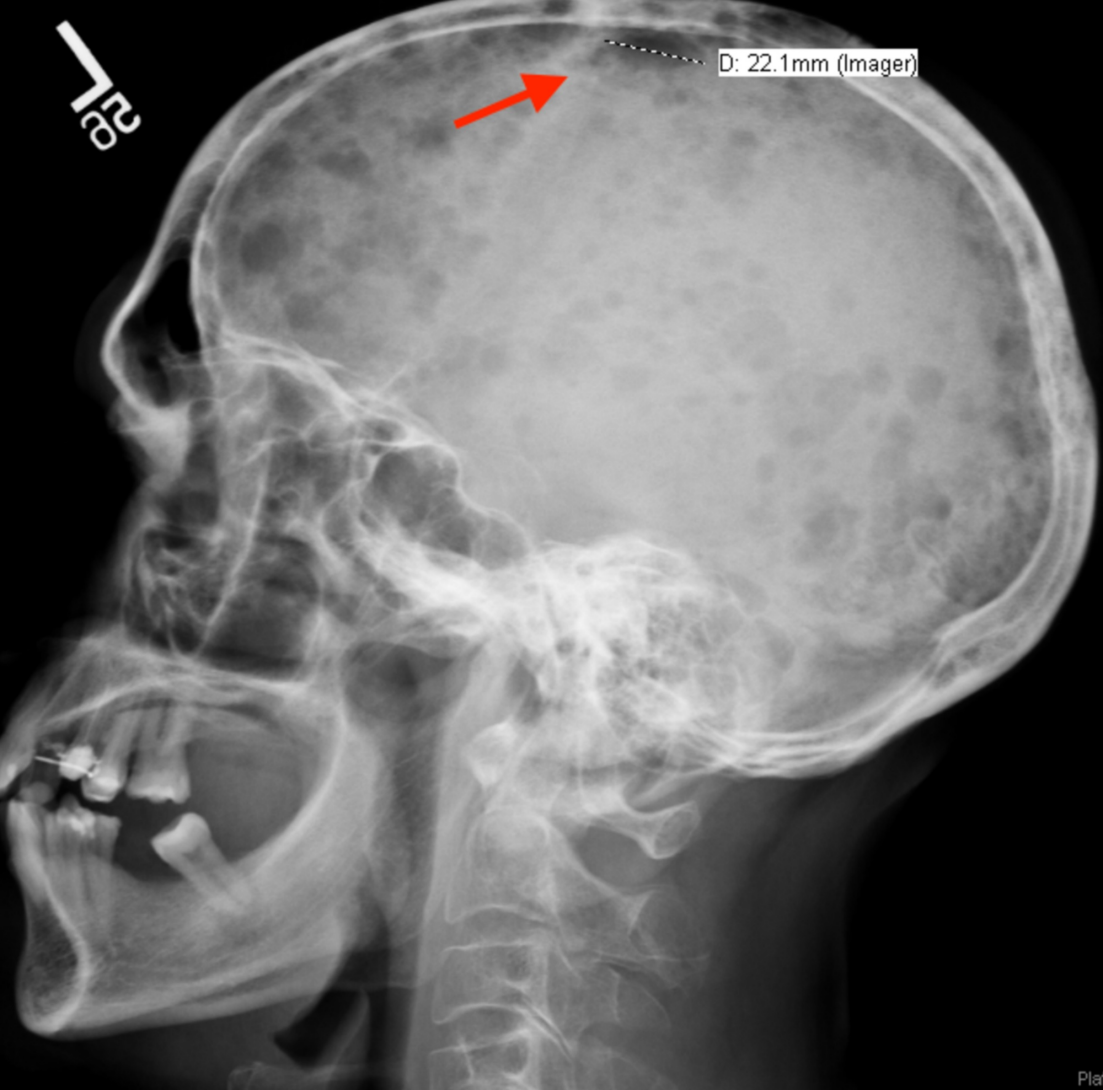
X-ray of the skull revealing a round, lytic lesion (red arrow), suggestive of multiple myeloma
